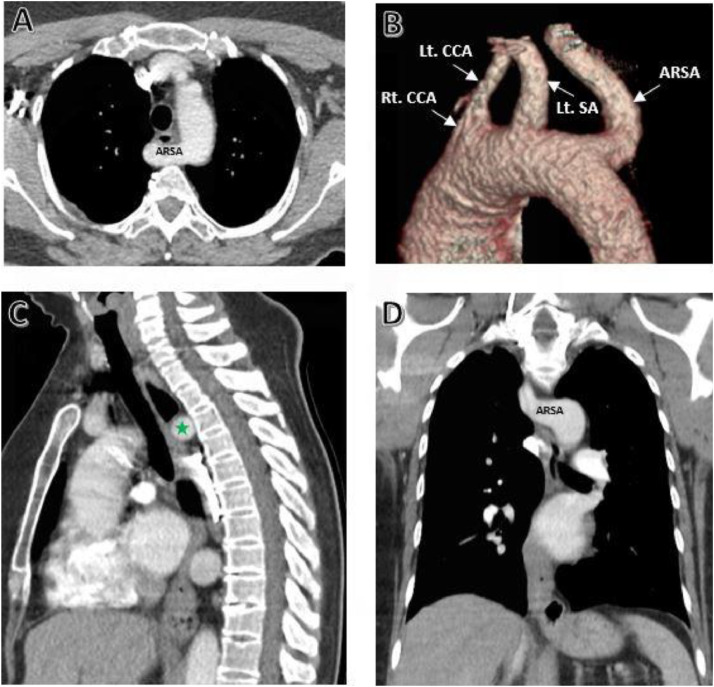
Contrast-enhanced CT scan images demonstrating the aberrant course of the right subclavian artery passing posterior to the mid-esophageal segment (green star). This vascular trajectory is consistent with the typical appearance of a right-sided aortic arch and aberrant right subclavian artery (ARSA). Image views: A) Axial B) Three-dimensional reconstruction C) Sagittal D) Coronal - ARSA, aberrant right subclavian artery; Rt. CCA, right common carotid artery; Lt. CCA, left common carotid artery; Lt. SA, left subclavian artery.
